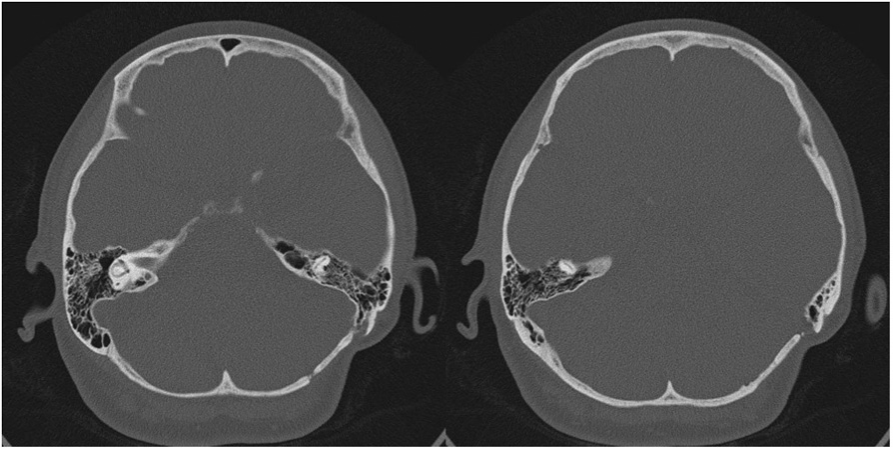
Preoperative bone-window computed tomography scan 4 years after first operation demonstrates nearly complete ossification.
